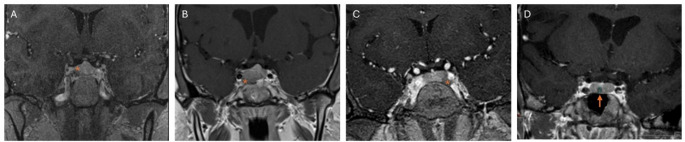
(A-C) Representative post-contrast magnetic resonance imaging (MRI) studies of pituitary corticotropinomas in three patients with Cushing’s disease and *USP8* pathogenic variants showing larger tumors with signs of cavernous sinus invasion (asterisk). (D) Representative post-contrast MRI study of a patient with Cushing’s disease without *USP8* pathogenic variant showing a typical microadenoma (arrow)
